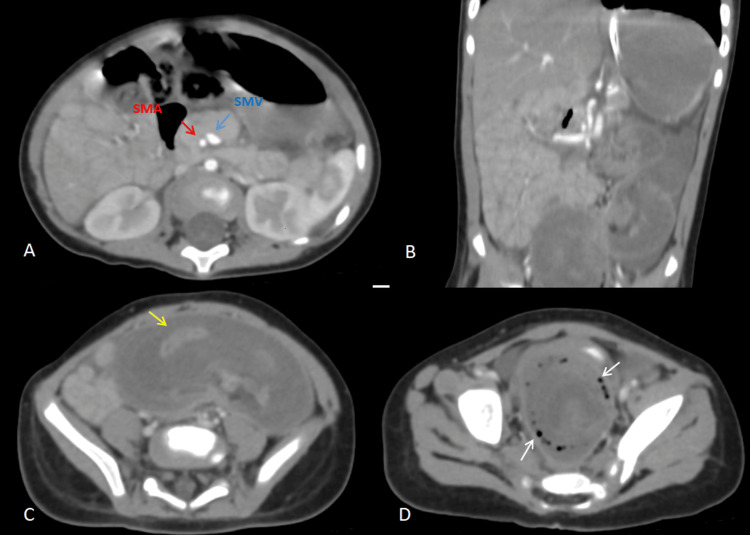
Contrast-enhanced CT of the abdomen. (A) The superior mesenteric vein is seen to the left of the superior mesenteric artery, indicating an abnormal superior mesenteric artery-superior mesenteric vein relationship. (B) Small bowel loops are predominantly on the right side, with large bowel loops on the left of the midline, consistent with intestinal malrotation. (C) Collapsed ileal loops with mesenteric fat and vessels telescoping into the lumen of the large bowel, representing ileocolic intussusception on the left side. (D) Thickened bowel loops with intramural air foci, suggestive of gangrenous changes. The red arrow depicts the superior mesenteric artery, the blue arrow depicts the superior mesenteric vein, the yellow arrow highlights Intussusceptum, and the white arrow highlights pneumatosis.
